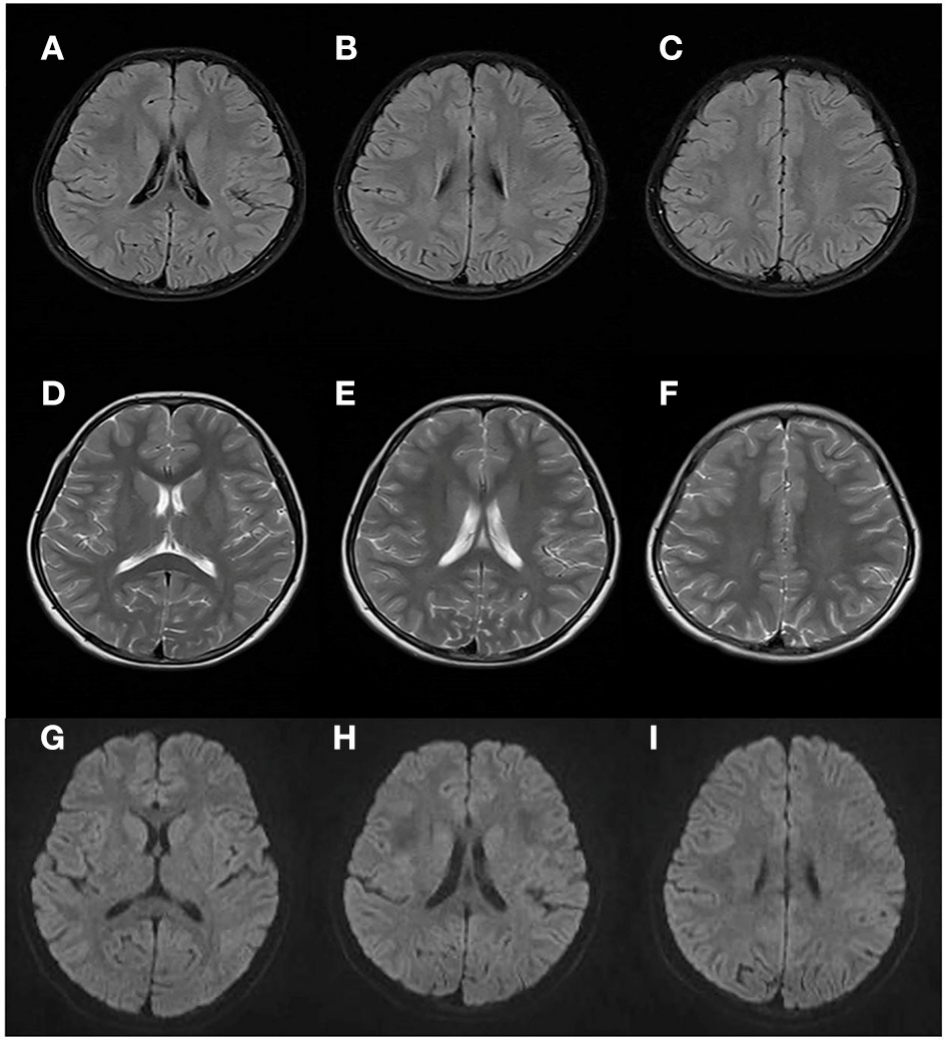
Brain MRI performed 45 days after the first attack. Results showed almost complete regression of the WM abnormalities (compared with Figure 1).
